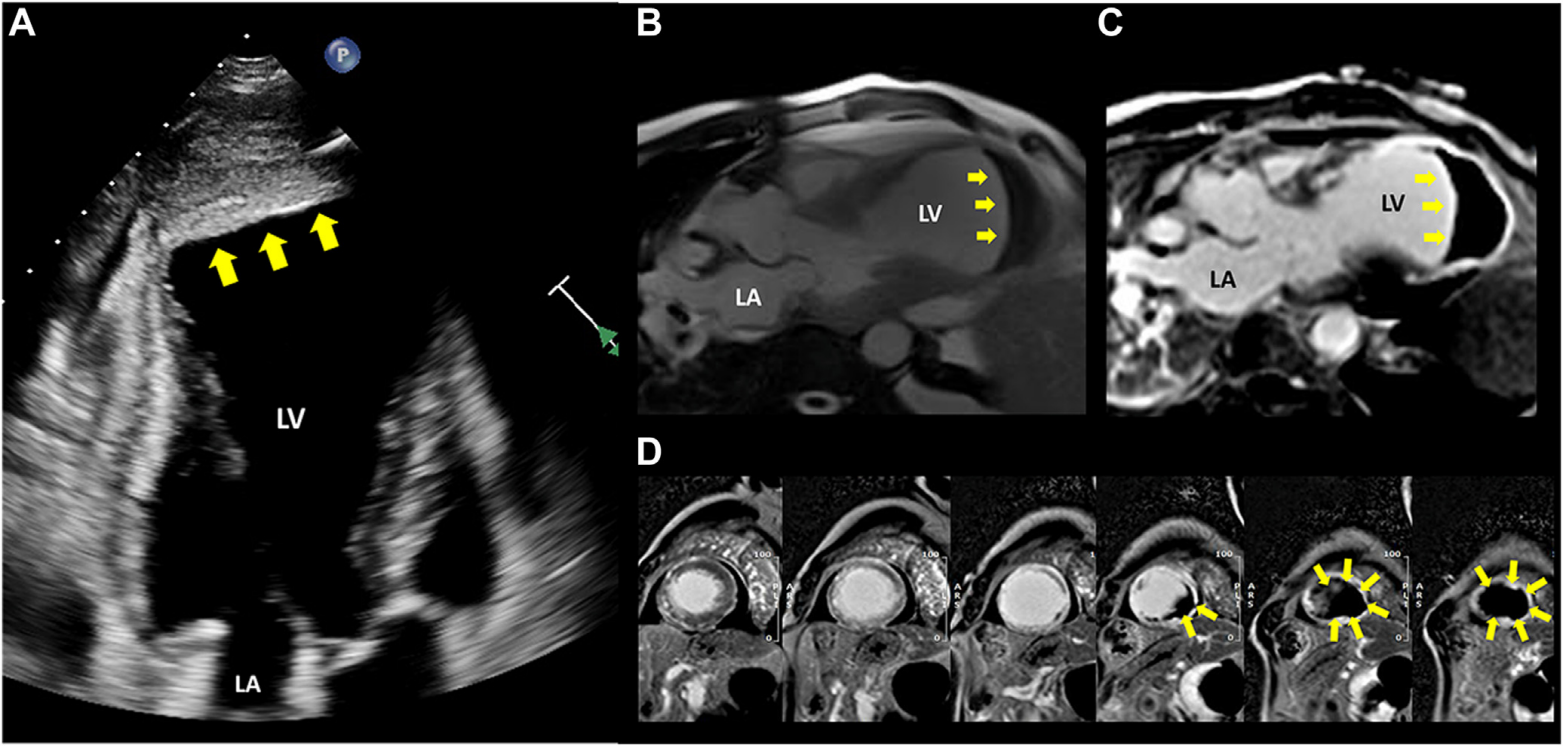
Transthoracic echocardiogram and cardiac magnetic resonance imaging at presentation. (A) Apical 3-chamber view on the transthoracic echocardiogram demonstrates a left ventricular (LV) apical aneurysm with substantial LV thrombus (yellow arrows). (B) Three-chamber steady-state free precession cine imaging showing a true LV apical aneurysm with a giant LV thrombus (yellow arrows) with indexed LV end-diastolic and end-systolic volumes of 201 mL/m^2^ and 179 mL/m^2^, respectively. (C,D) Late gadolinium enhancement imaging demonstrating the thrombus (yellow arrows) and transmural myocardial enhancement of the LV apex—(C) 3-chamber view; (D) short-axis views from mid-ventricular to apex. LA, left atrium; (in figure) LV, left ventricle.
